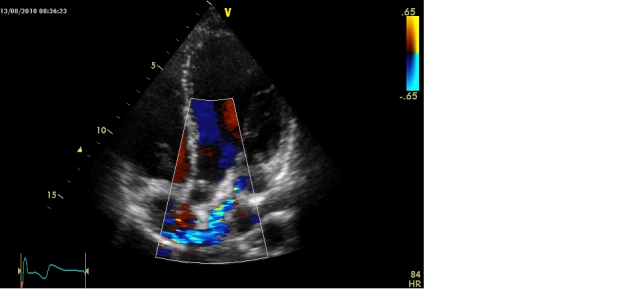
Coronary fistula between coronary left sinus and right atrium.Transthoracic 2D echocardiography - apical 5 chambers –reveal a tortuous ductus that overlaps the image of the left atrium and flows into the right atrium.
